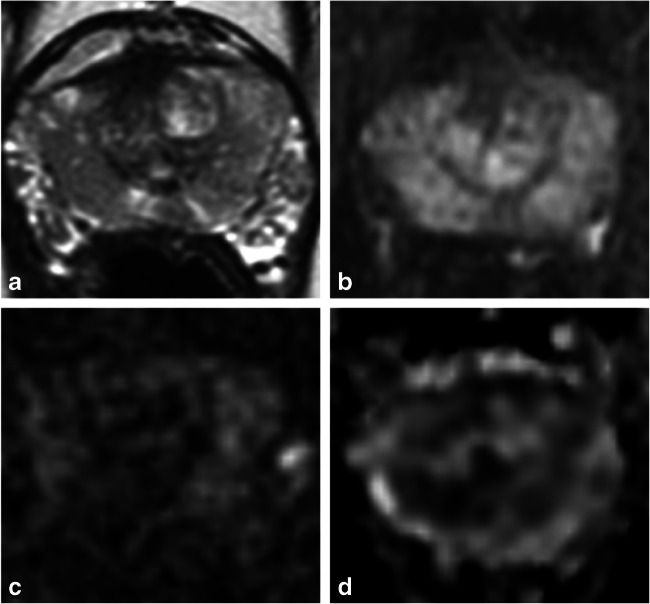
A 44-year-old man with a family history of prostate cancer, PSA 0.5 ng/mL and subsequent negative transrectal-ultrasound-guided biopsy. T2-weighted (a), early-phase dynamic contrast-enhanced (b), b-2000 diffusion-weighted (c), and apparent diffusion coefficient map (d) images show features of score 4 for each of the individual sequences
